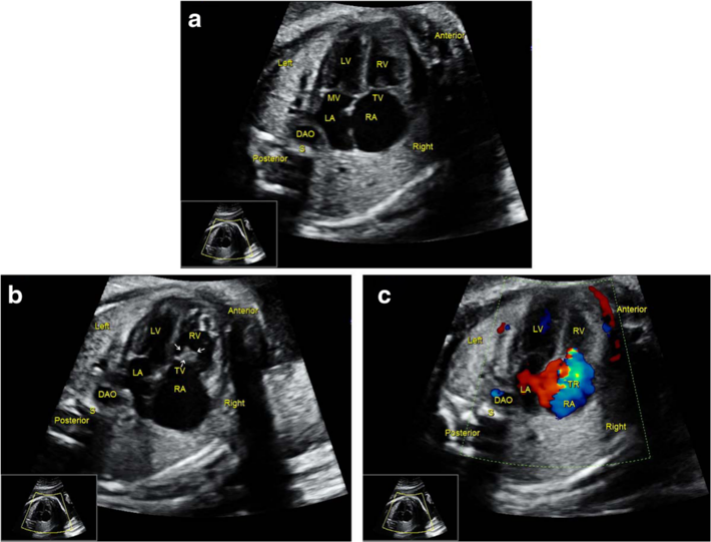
Sonographic images of a 24-gestational-week fetus diagnosed with tricuspid dysplasia. The 4CV was considered to be normal by sonographers under Protocol A because they did not find a thickened tricuspid valve (a). In fact, the RA was a little large in size according to careful observation. Also, the thickened tricuspid valves could be detected after careful scan (b). Color Doppler made it quite easy to detect tricuspid regurgitation, which would direct attention to valvular structural anomalies (c). DAO, descending aorta; LA, left atrium; LV, left ventricle; RA, right atrium; RV, right ventricle; S, spine; TR, tricuspid regurgitation; TV, tricuspid valve. The *arrows* indicate the thickened valves
